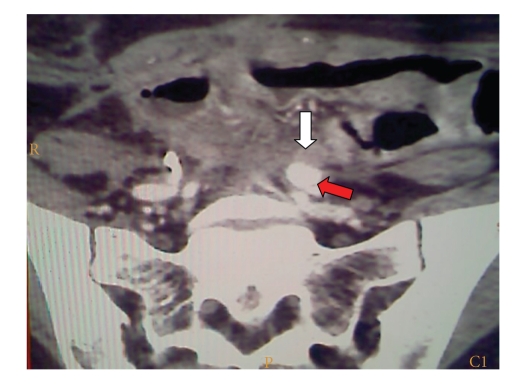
CT scan demonstrating left common iliac artery (red arrow) and left ureter (white arrow) which appear not dissociable.
